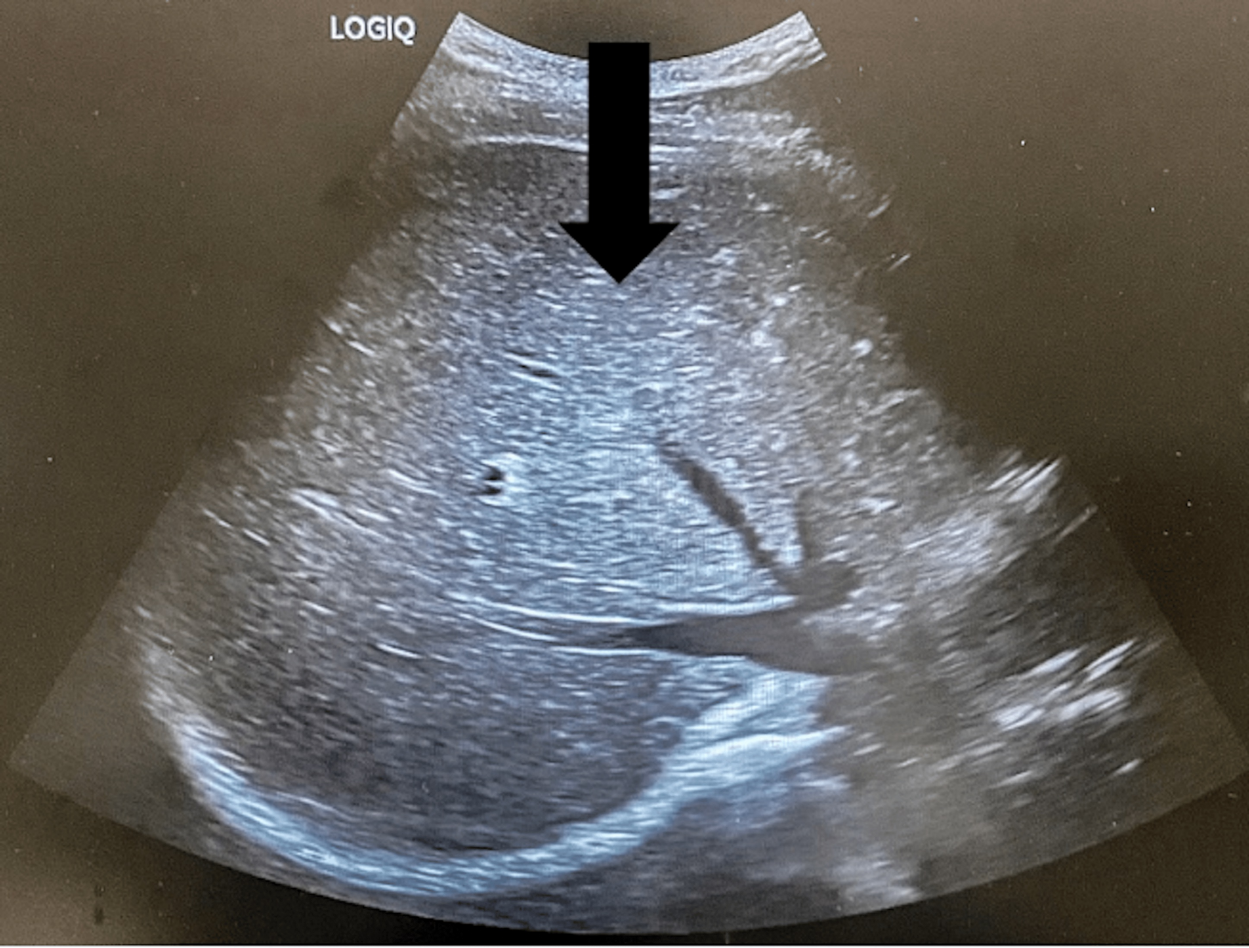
The abdominal cavity ultrasonography showing a hyperechogenic liver of average size (arrow).
